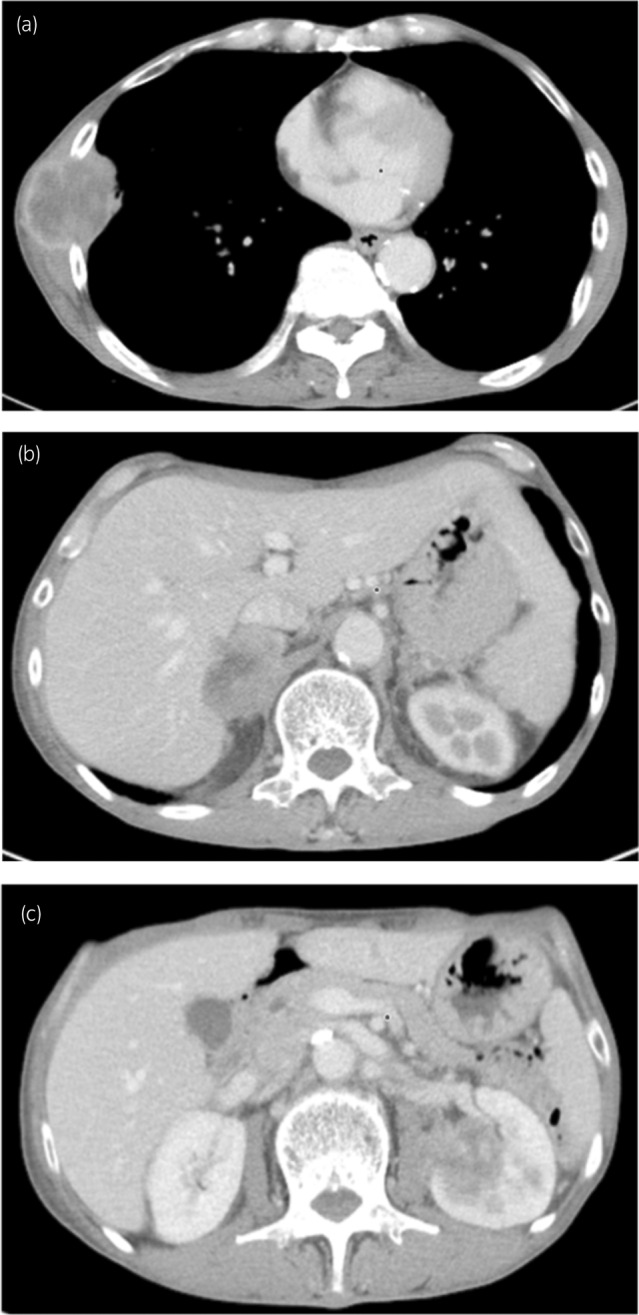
CT image on admission. (a) A 4 × 4 cm mass is visible on the right thoracic wall. (b) A mass is seen on both adrenal glands. (c) A tumor with contrast enhancement and irregular margins is visible on the left kidney.
